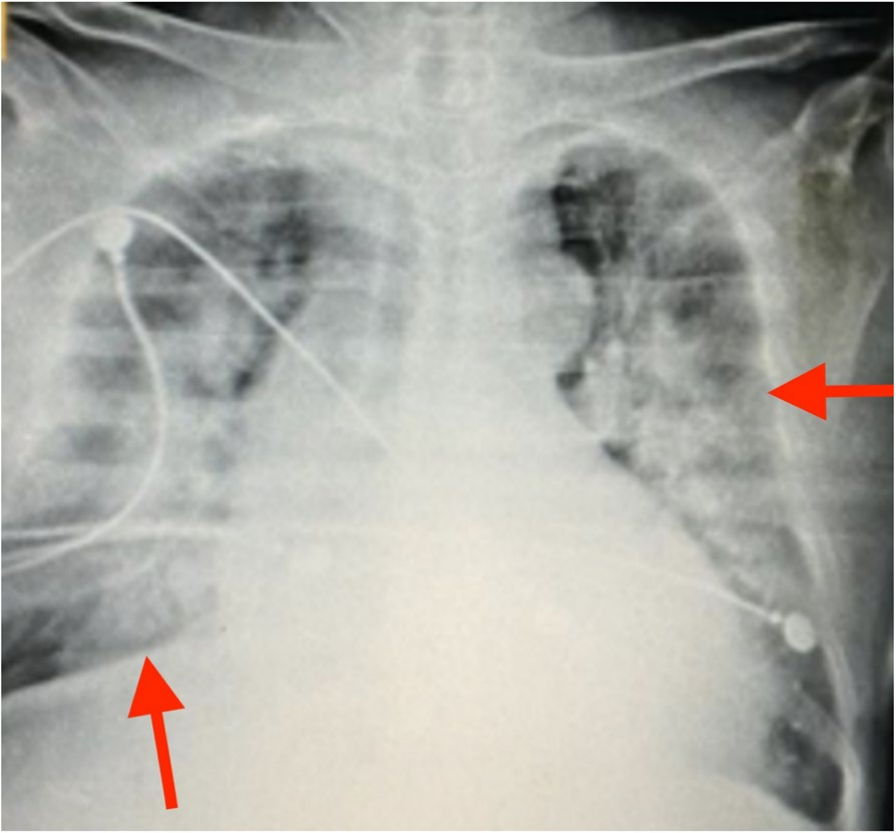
Portable chest radiograph showing multiple radiopacities of interstitial occupation and peripheral distribution (red arrows)
